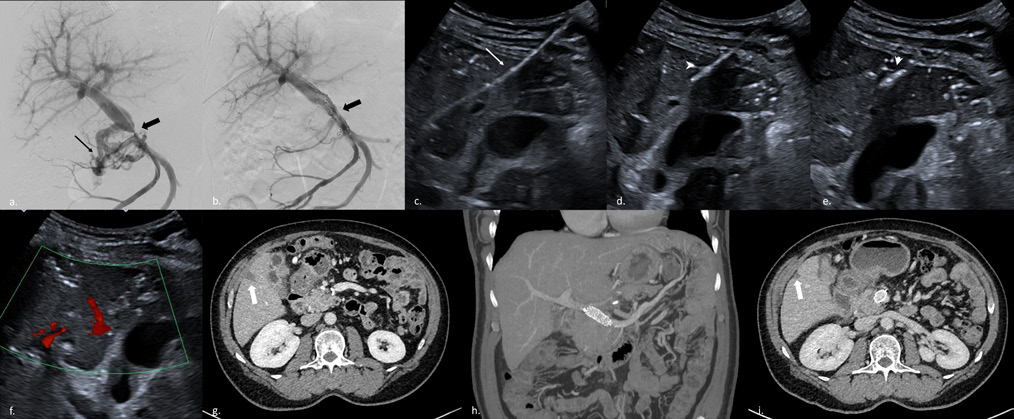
Pre- and post-operative aspect a. Severe portal stenosis (black thick arrow) with collateral pathways (black arrow) b. Stent was deployed across the stenotic segment (black thick arrow) allowing satisfactory portal flow and disappearance of collateral pathways. c. Visualization of the puncture tract with the sheath (thin arrow) under ultrasound. d and e. Microwave probe (arrowhead) is inserted along the puncture tract and thermal-ablation is performed f. Ultrasound control using Doppler showed the absence of active bleeding and a patent portal vein g. First scan after intervention showed a sub capsular hypodense triangular patch corresponding to ablation zone (thick arrow) h. Second scan performed 5 months later with MIP reconstruction: patent stent without deviation route i. 5 months later, capsular retraction and decrease in the triangular patch (thick arrow).
